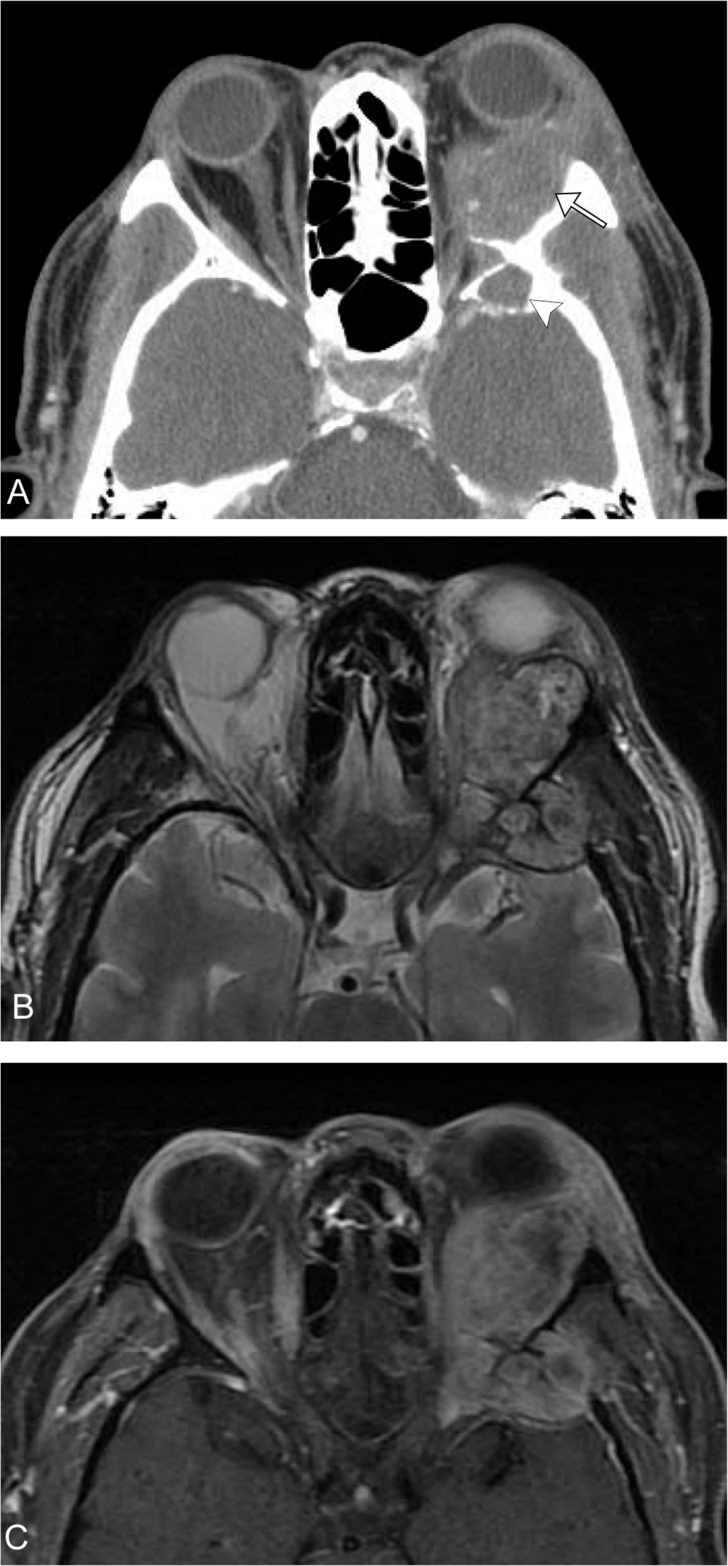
Epithelioid hemangioma. A 55-year-old male, with gradual left eye proptosis. a Axial CT demonstrates a multinodular mass centered along the lateral margin of the left orbit (arrow), also involving the left sphenoid wing (arrowhead). b Axial T2 image demonstrates the mass to be heterogeneous in appearance. c Axial T1 post-contrast fat-suppressed image demonstrates heterogeneous enhancement. These imaging features are non-specific. The patient underwent biopsy, which revealed epithelioid hemangioma
